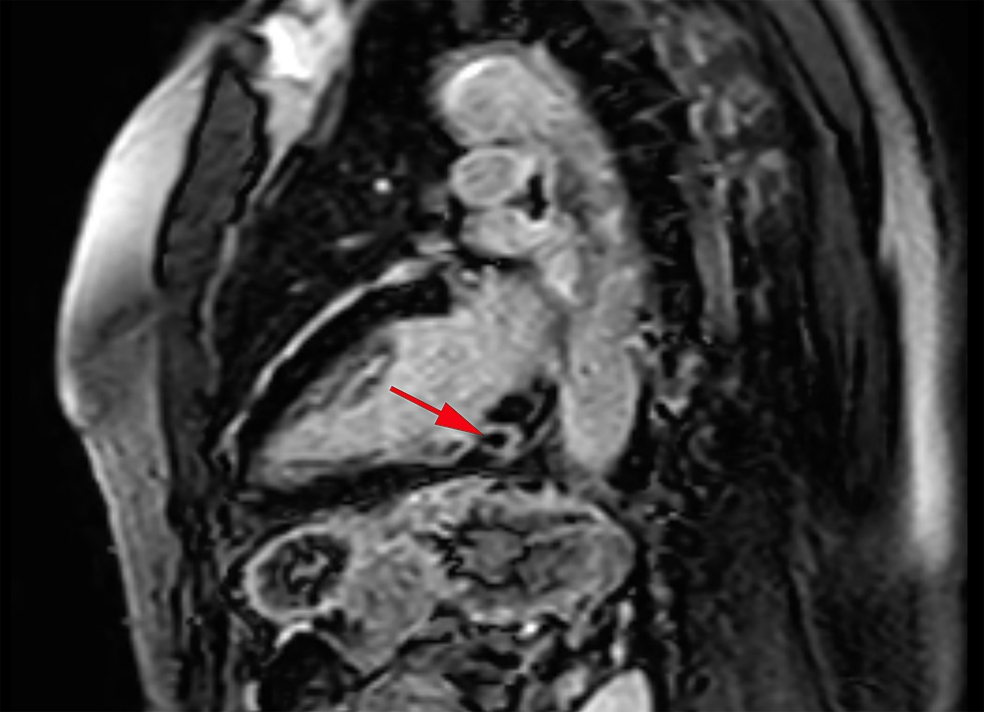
Cardiac MRI. Cardiac MRI showing near-transmural delayed enhancement and nulled area within the delayed enhancement in the basal inferior wall due to a recent ablation procedure. Red arrow points to the nulled area. MRI: magnetic resonance imaging
